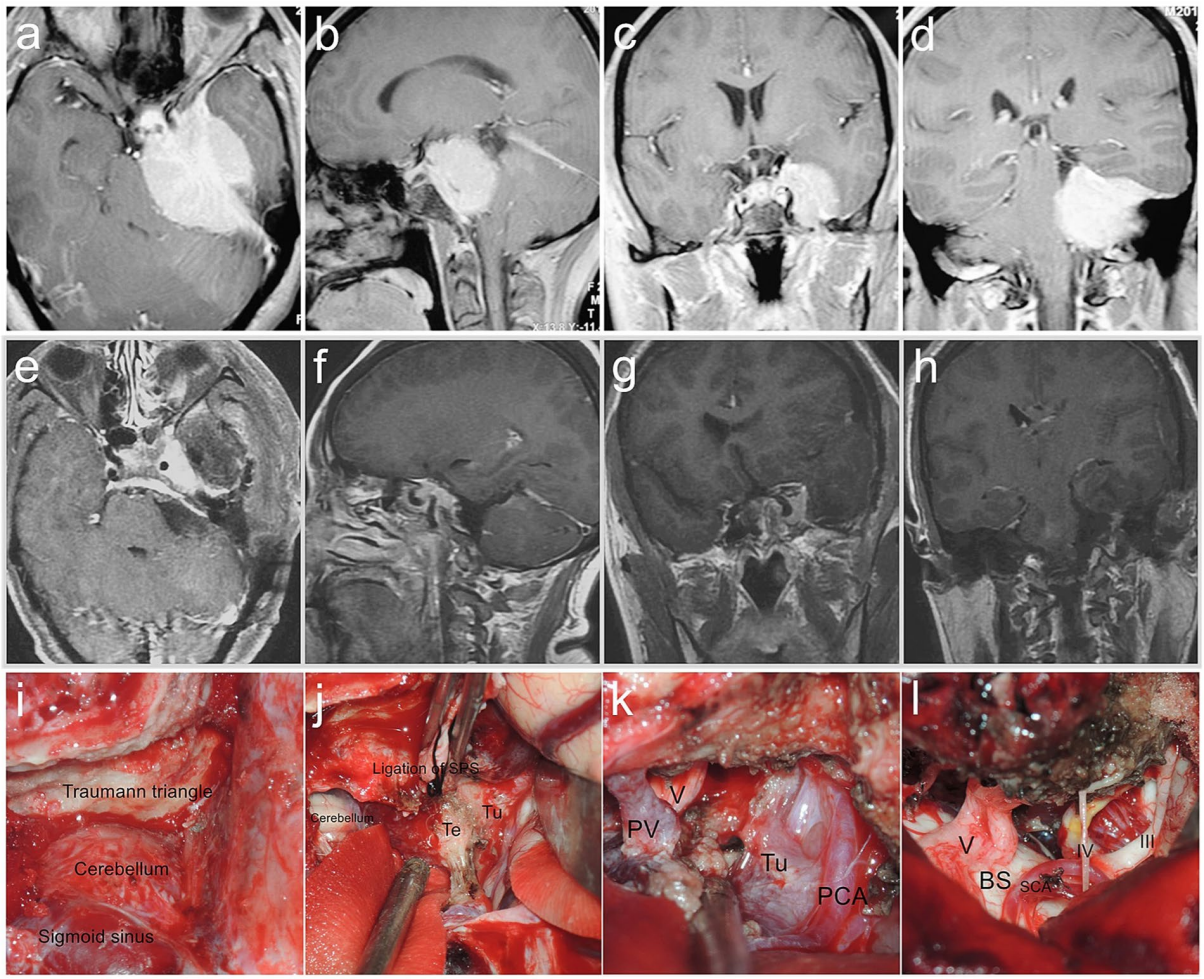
Petroclivosphenoidal type case 3. A 44-year-old male presented with headache prosopalgia and hobble for 18 months. The preoperative KPS score was 70. He was achieved STR with the PCA of palsy in CN V, VII and VIII. The postoperative KPS score was 50. And he received the GKS after discharge of 1 month. (a–d) Preoperative MRI T1 contrast axial, sagittal, and coronal images. (e–h) Postoperative MRI T1 contrast axial, sagittal, and coronal images. (i–l) Intraoperative figures demonstrating steps of petroclivosphenoidal type resection via the PCA. (i) Exposure of the traumann triangle, cerebellum, sigmoid sinus and transverse sinus. (j) Ligation of the superior petrosal sinus and incision of the tentorium to expose the supra-infratentorial structures and tumor. (k) Tumor resection between the multiple intervals of the neurovascular structures. (l) Subtotal tumor resection and keeping well the brain stem and neurovascular structures integrity. SPS, Superior Petrosal Sinus; SCA, Superior Cerebellar Artery; PCA, Posterior Cerebral Artery; Tu, Tumor; PV, Petrosal Vein; Te, Tentorium; BS, Brainstem.
